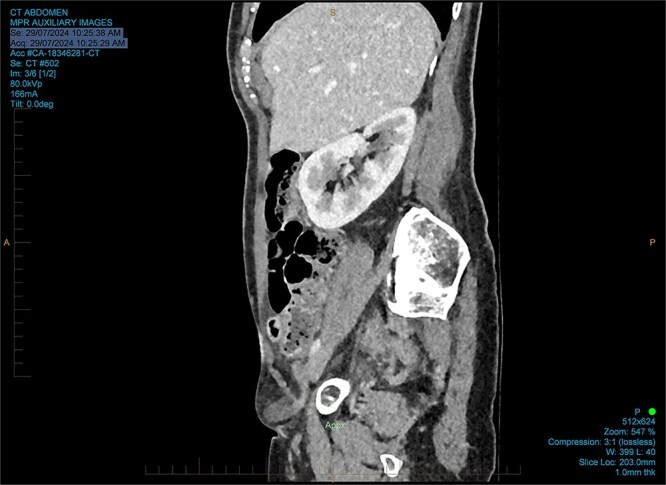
Sagittal CT reported as an Amyand’s hernia.
